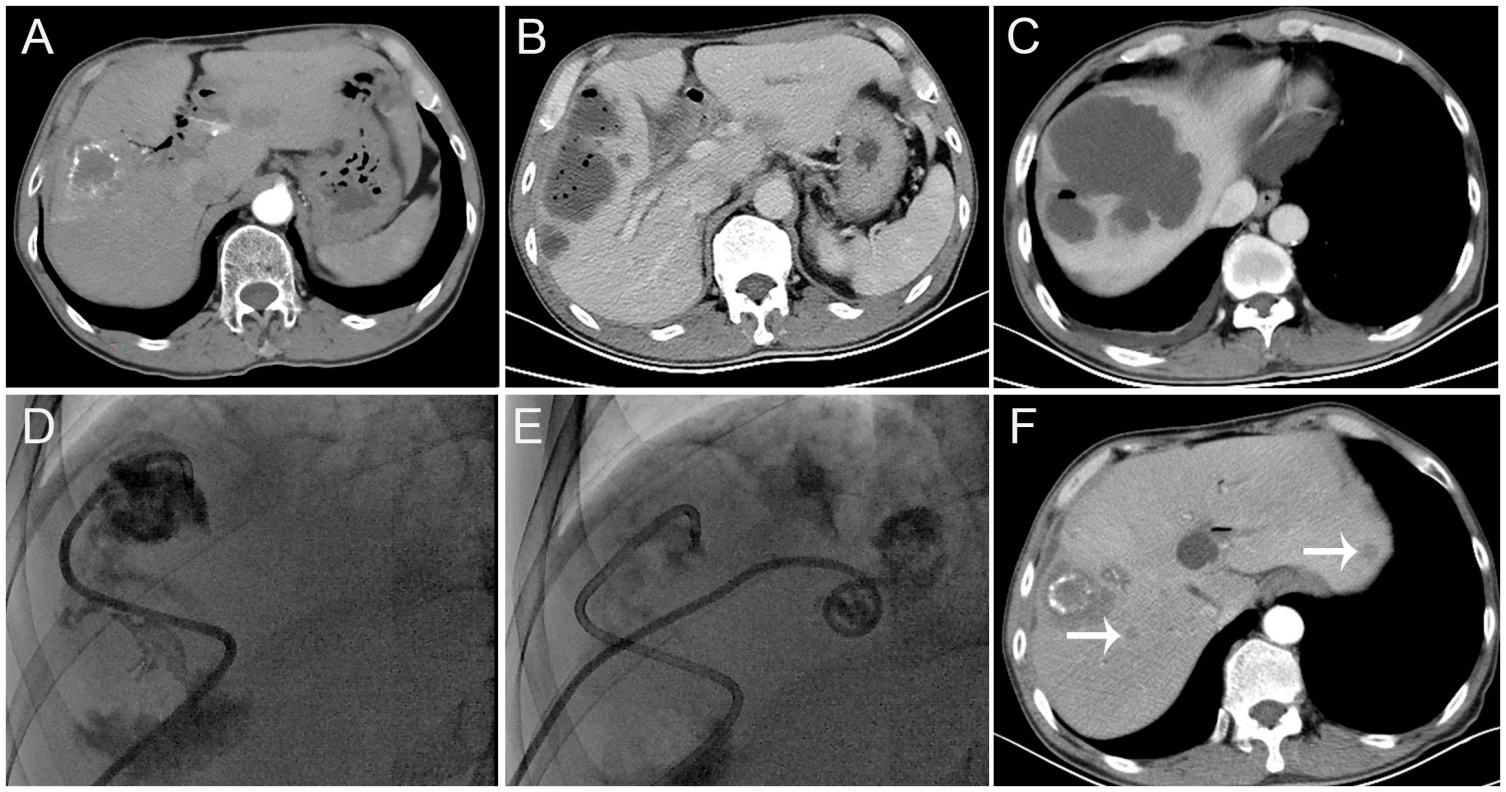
Findings from a 62-year-old patient with liver metastasis of cholangiocarcinoma and a history of biliary-enteric anastomosis and one previous transarterial chemoembolization (TACE) session. (A) Before the TACE session, contrast-enhanced computed tomography scans revealed hypodense lesions in segment V of the liver, with no significant enhancement. A small amount of iodized-oil emulsion deposit was observed, and the intrahepatic bile duct showed multiple pneumobilia and dilatations. (B, C) Thirteen days after this TACE session, contrast-enhanced computed tomography scans revealed the formation of multiple gas-containing liver abscesses. (D, E) Subsequently, the patient underwent percutaneous drainage (PCD). (F) One month after PCD, contrast-enhanced computed tomography scans showed an increase in tumor size and multiple new metastases in the liver (arrow).
